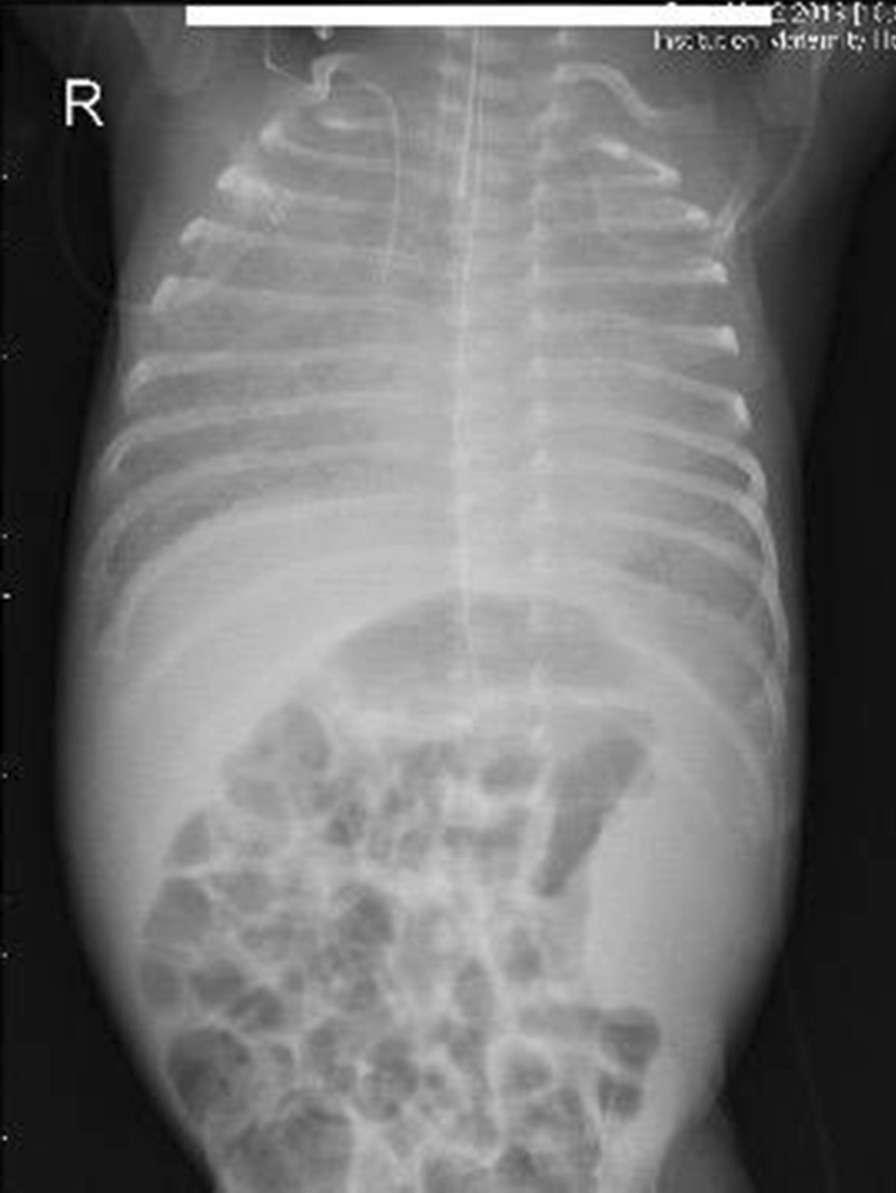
Patient’s chest X-ray on admission
